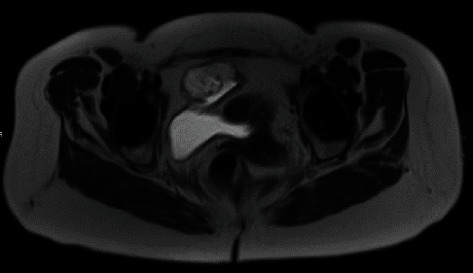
Magnetic resonance imaging showing a lesion at the expense of the bladder wall.
